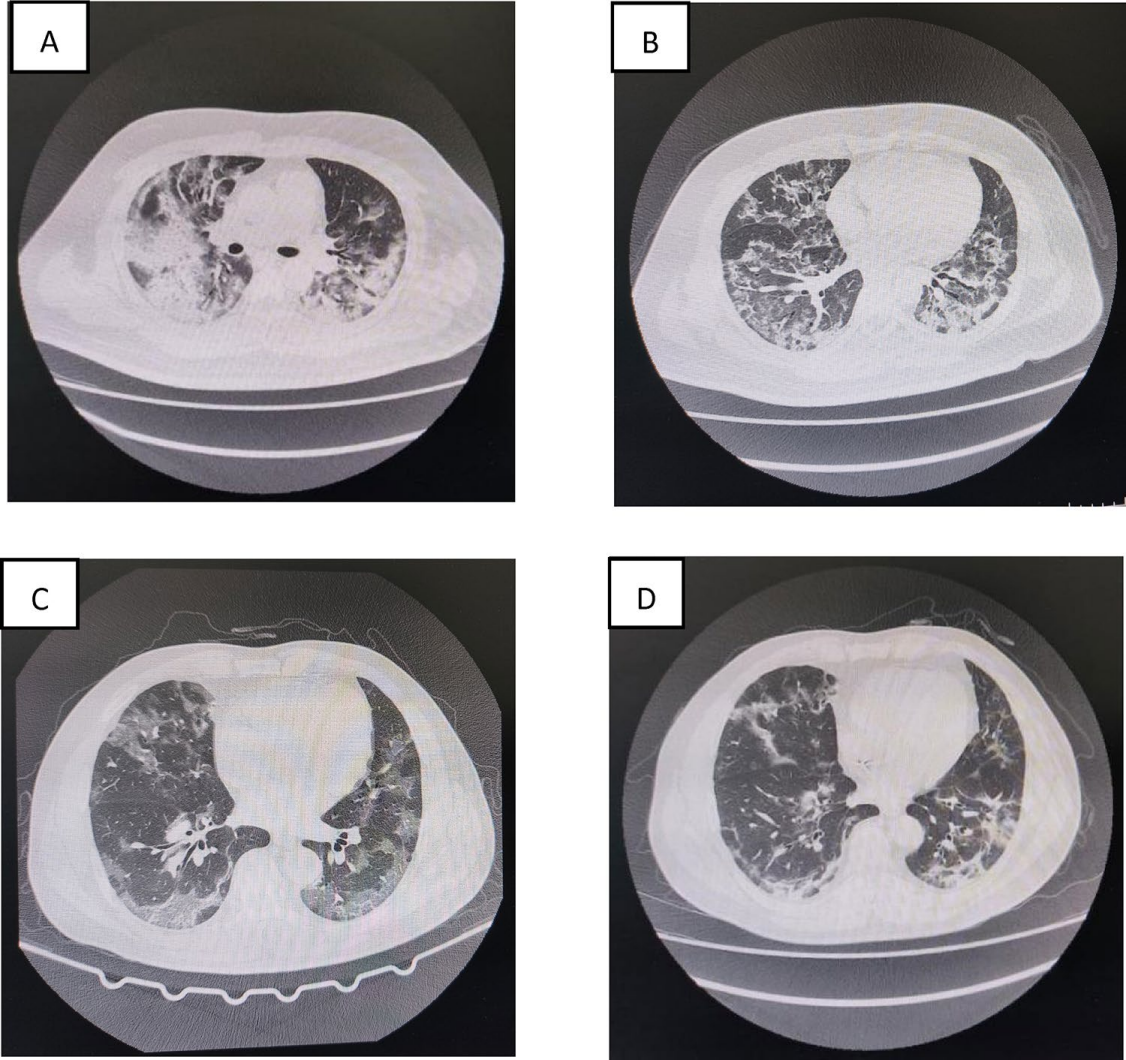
Chest computed tomographic scans in the two severe COVID-19 patients infected with the delta variant of SARS-CoV-2. Chest computed tomographic (CT) scan taken before prone position (PP) in case 1. B CT scan taken on after PP in case 1. It revealed the lung infiltrates improved significantly compared with A. C CT scan taken before PP in case 2. D CT scan taken after PP in case 2. It revealed the lung infiltrates improved significantly compared with D
